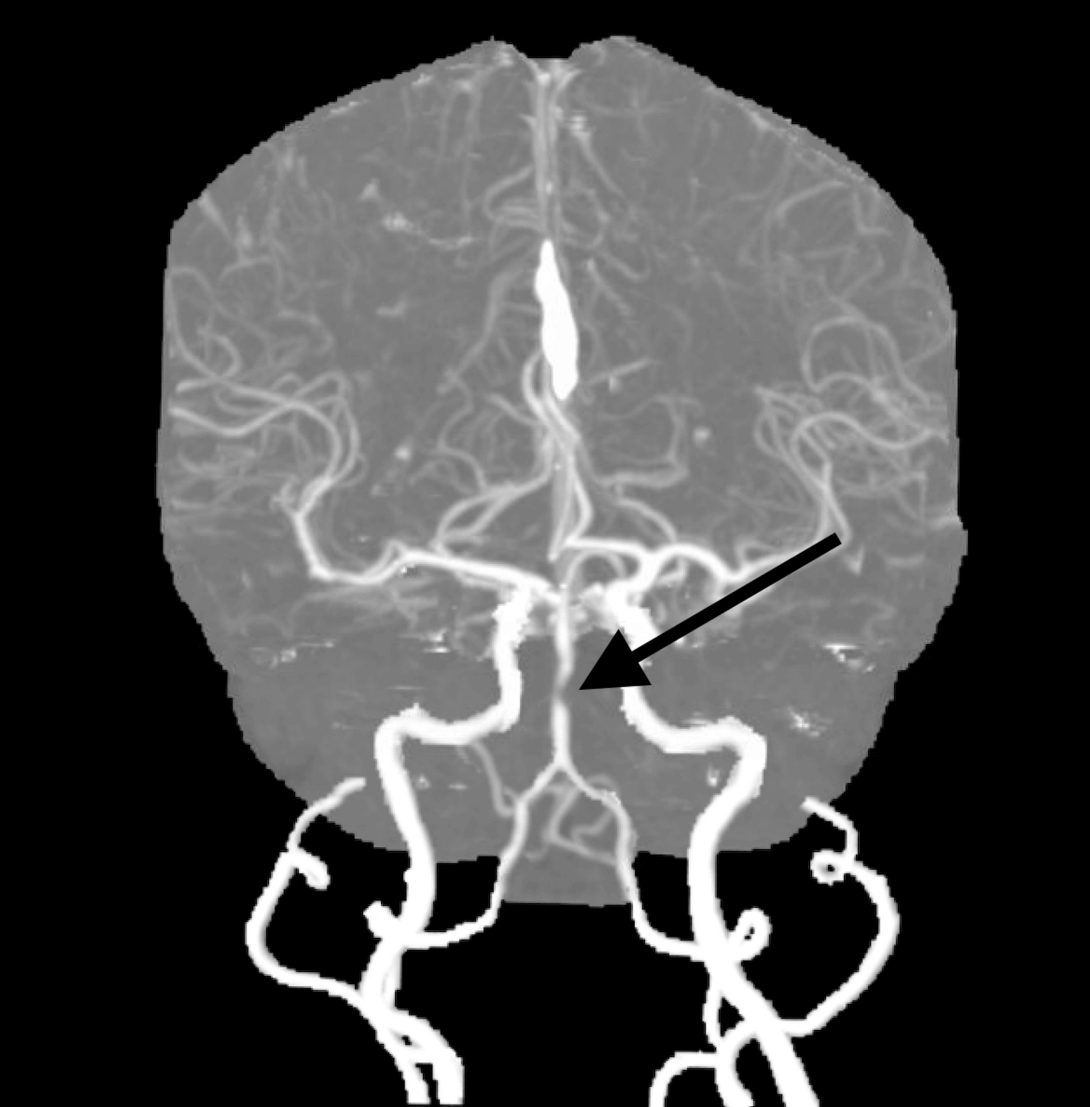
CT angiogram revealed a high-grade mid-basilar stenosis (indicated by the black arrow).
